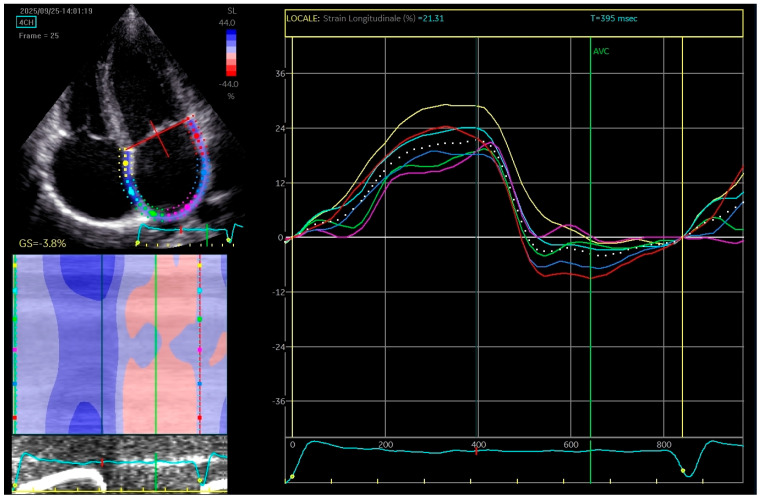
The apical four-chamber view was utilized for the strain measurements of the left atrium (LA); the edge of the LA endocardium was manually traced. The software generated tracings based on the 2D strain of LA. The mean deformation (strain) is expressed in percentage and calculated by the software.
